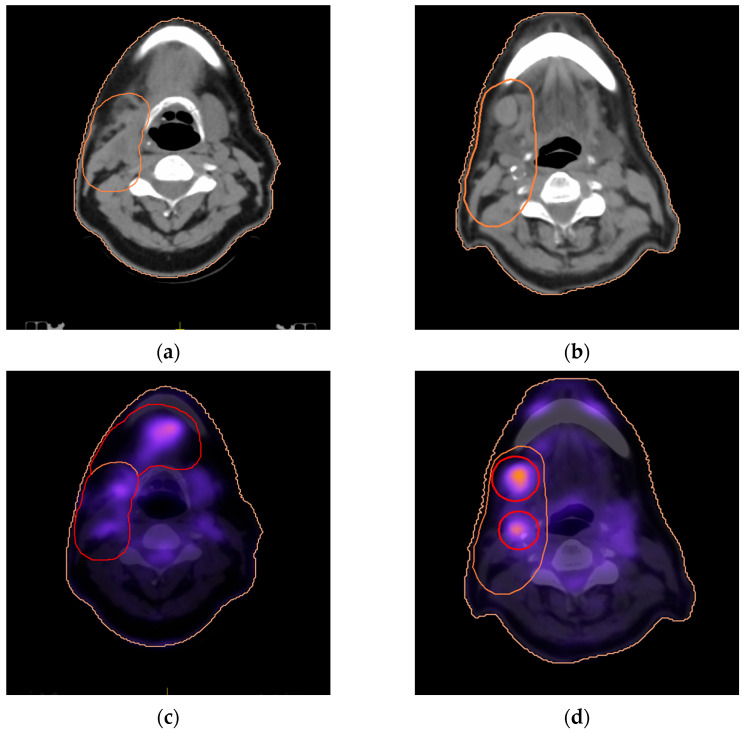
Examples of PET/CT-guided radiotherapy planning showing extended PTV2 area to cover submandibular extension undetected in the CT scan (orange line extended to red line) (a,c). Delineation of metabolically active nodes to receive a booster dose within the PTV2 area (b,d).
